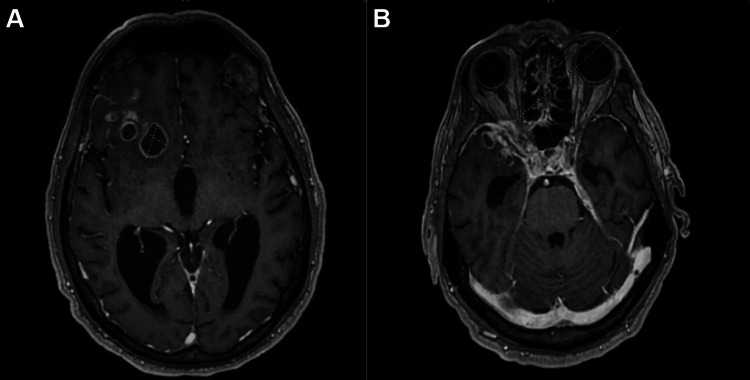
MRI of the brain confirming multiple brain abscesses. Panel (A) shows the right frontal lobe abscesses with adjacent leptomeningeal thickening (2.1 cm). Panel (B) highlights a subperiosteal abscess in the posterior right orbit with mild optic nerve mass effect. MRI: Magnetic resonance imaging
